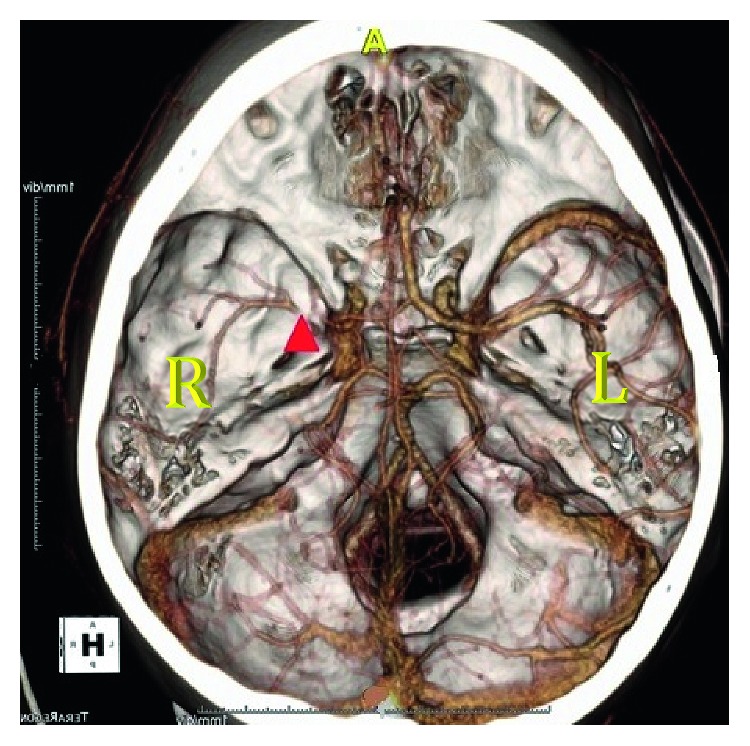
3D reconstruction of the CTA showing stenosis or embolus in the right MCA.
